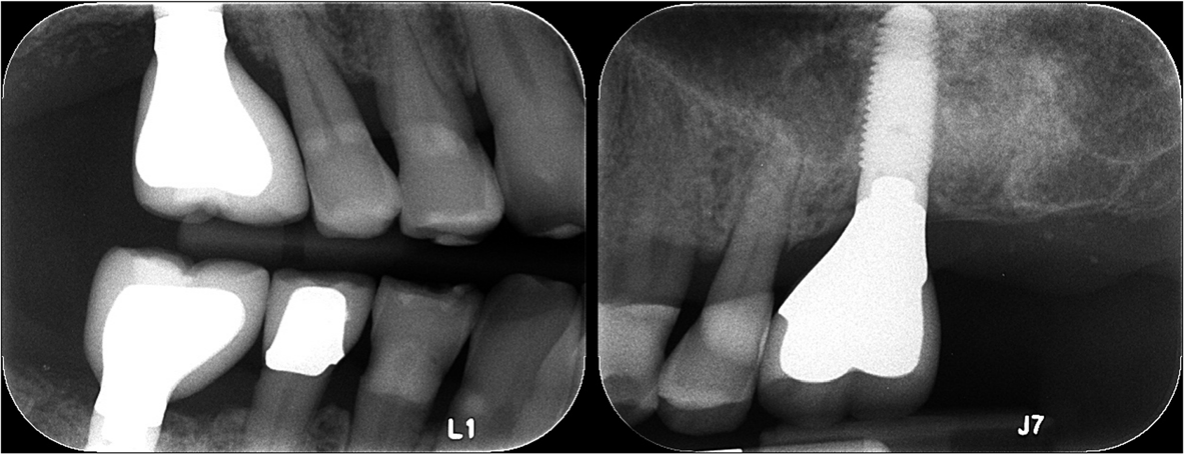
Radiographic bone levels three years after placement. Bone levels remain unchanged during long-term follow-up
